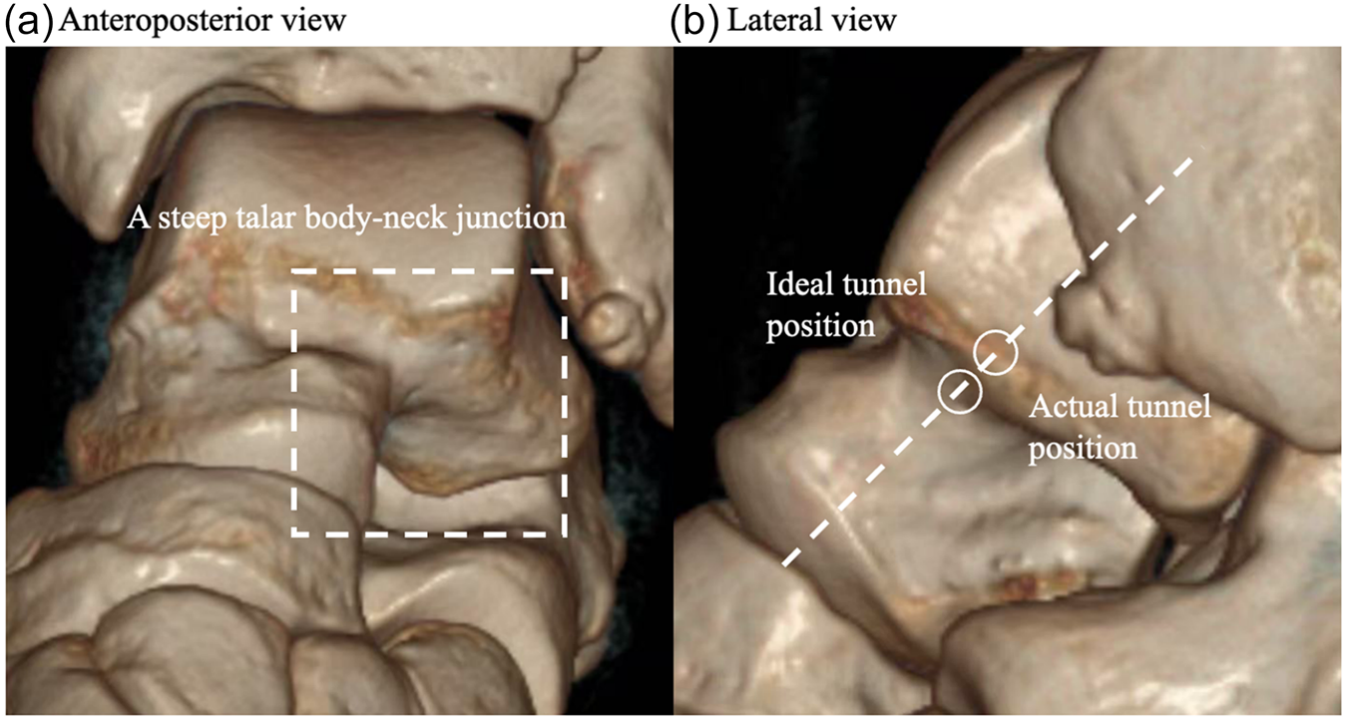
The (a) anteroposterior and (b) lateral 3D‐CT views of a CLAI patient with steep talar body–neck junction. Under this circumstance, drilling at the ideal tunnel position is relatively unstable and might lead to a short talar tunnel. Therefore, the talar tunnel was after a horizontal posterior displacement of the tunnel along the long axis of the talus onto the talar body, which resulted in a lowered position on the ALTP‐ACT line. 3D‐CT, three‐dimensional computed tomography; ACT, anterolateral corner of the trochlea; ALTP, apex of the lateral talar process; CLAI, chronic lateral ankle instability.
